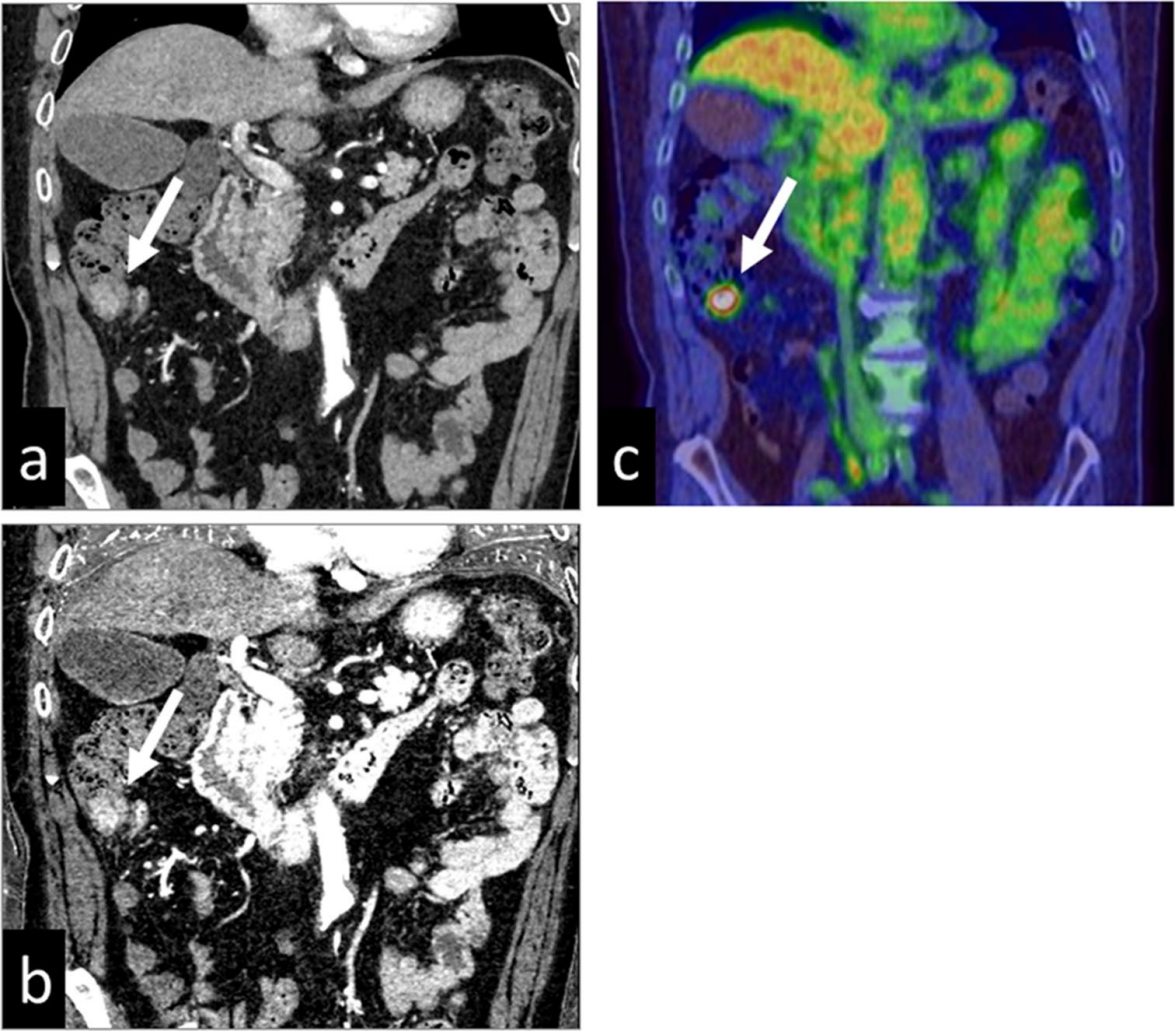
A 71-year-old man with cancer of the ascending colon. Virtual monochromatic image at 70 keV (a) and iodine map (b) during the arterial phase are shown. The iodine map yields better conspicuity than the monochromatic 70 keV image. c PET-CT image (the maximum standardized uptake value of the tumor was 6.1)
